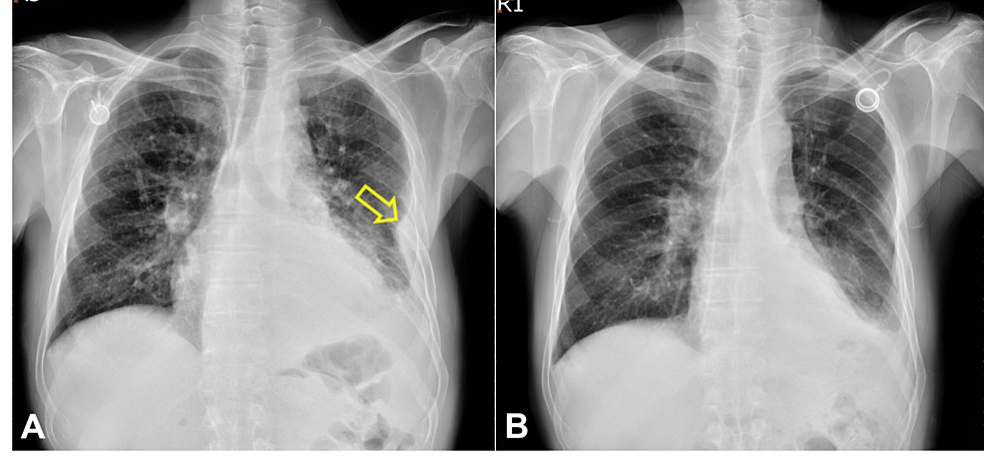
Chest X-ray films of the patient at the initial stage of treatment . A. After removal of the pigtail drainage catheter five days prior to the first course of cisplatin/paclitaxel chemotherapy (Jun. 8, 2020). Yellow arrow: pleural tumor seeding. B. Reimplantation of a Port-A catheter due to malfunction of the old one 25 days after the first course of cisplatin/paclitaxel chemotherapy (Jul. 8, 2020). Very good response in pleural seeding and effusion could be seen.
